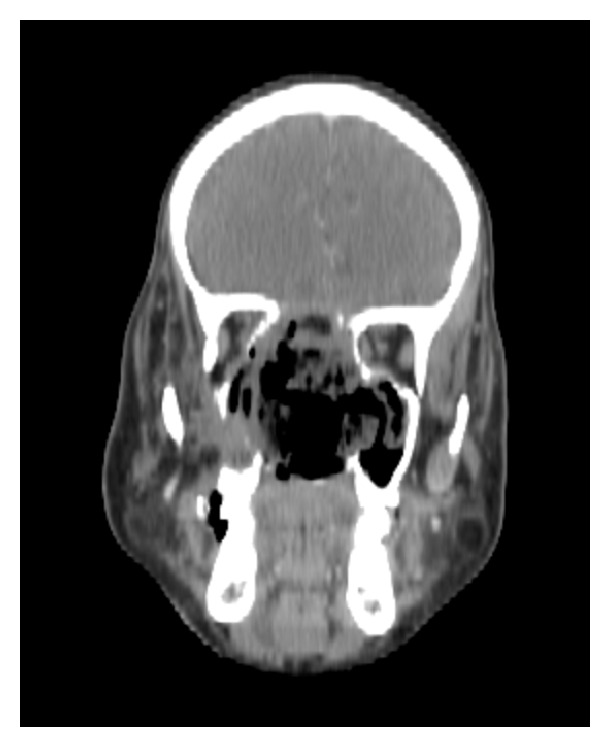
Coronal cut of contrast enhanced CT PNS showing postoperative changes and no recurrence.
